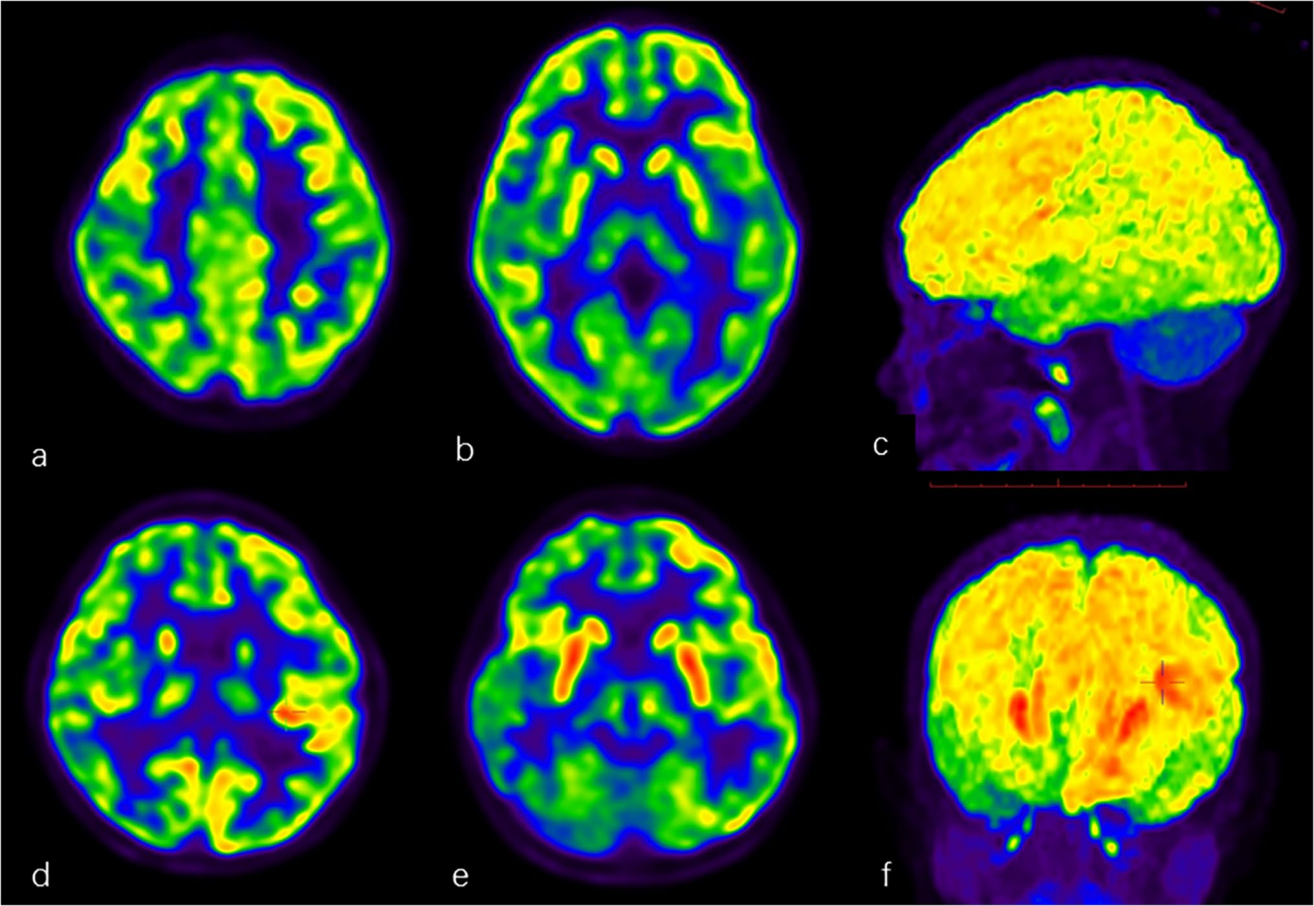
Top row: A 4-year-old girl presenting with fewer words and personality changes. Anti-MOG antibody test was positive. Brain ^18^F-FDG PET/CT showed large-scale lobar hypometabolism in bilateral parietal and occipital lobes without focal hypermetabolism (a, b, c). Bottom row: An 8-year-old girl presented with seizures, personality, and cognitive changes for more than 3 months, who was finally diagnosed with anti-NMDAR AE. Brain ^18^F-FDG PET/CT showed large-scale hypometabolism in bilateral parietal, occipital, temporal, and right frontal lobes (d, e, f) with focal hypermetabolism in left frontal and fronto-temporal lobe (the cross: d, f) and bilateral basal ganglia (e, f). c, f MIP map of FDG PET
